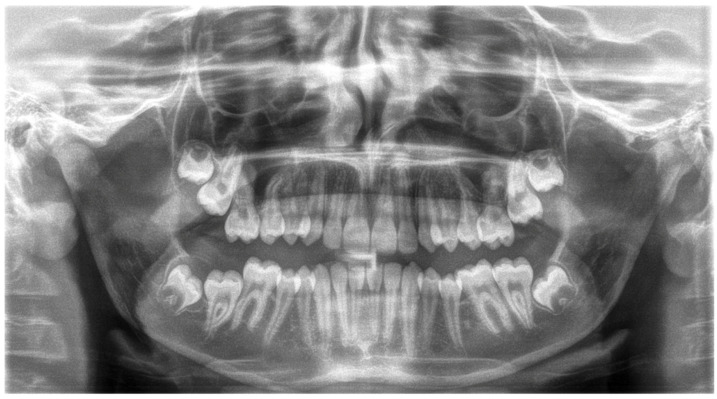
Orthopantomographic analysis.
